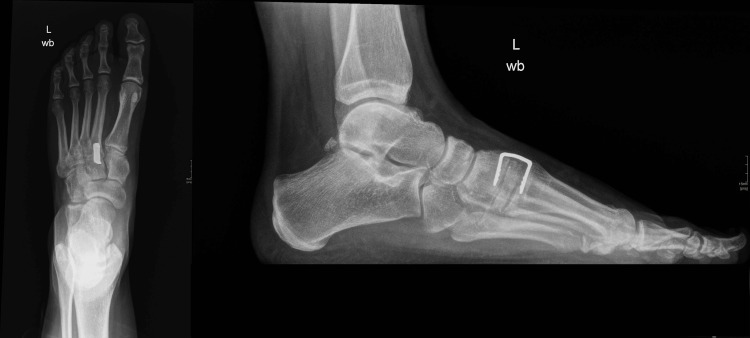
Postoperative weight-bearing radiographs of midfoot arthrodesis using nitinol compression staples, demonstrating maintained alignment and low-profile fixation
